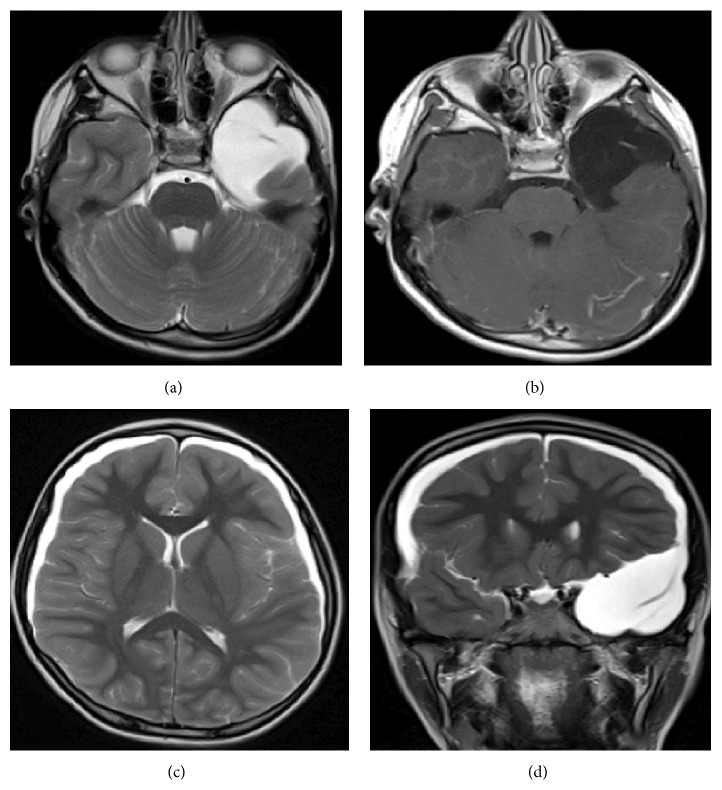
MRI brain axial T2 and T1 weighted postcontrast images (a) and (b) showing an extra-axial fluid signal intensity lesion in the left temporal region without any solid component or abnormal enhancement. Axial and coronal T2 weighted images show bilateral frontoparietal crescent shaped subdural fluid collections.
